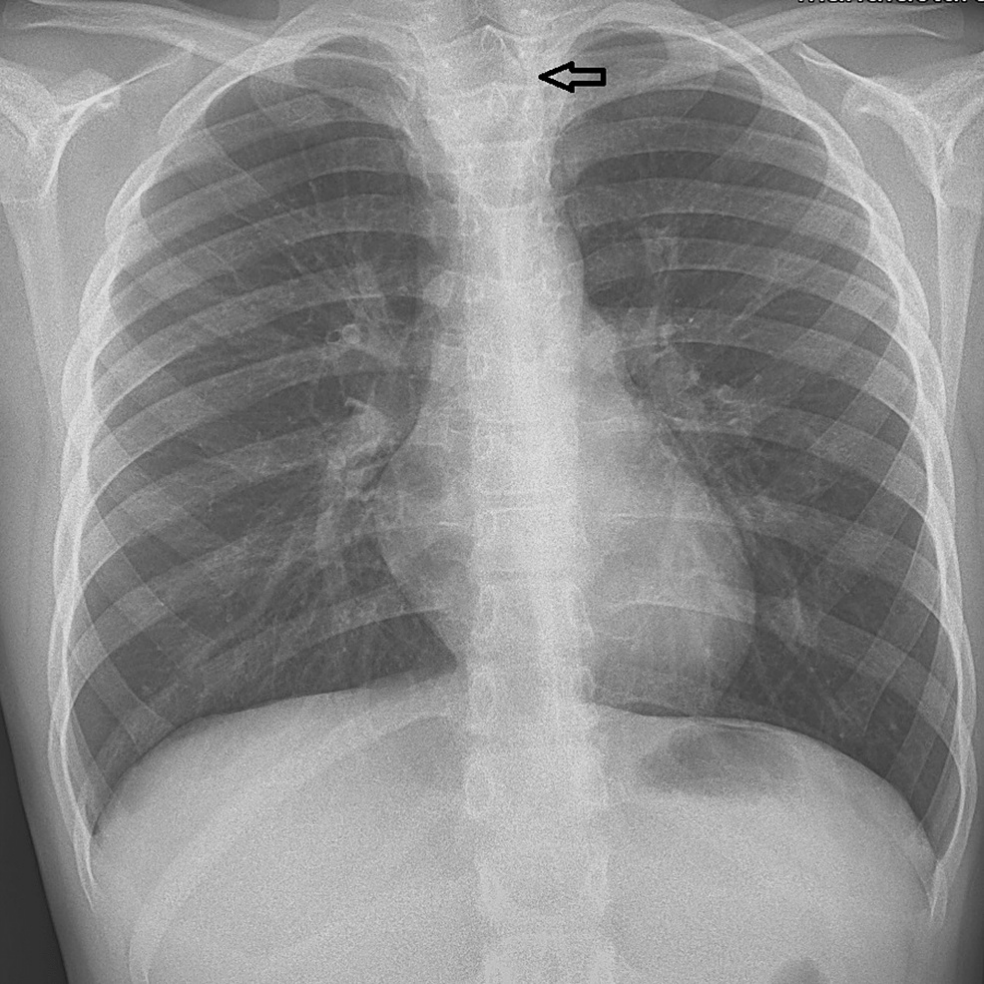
Chest X-ray A clear tracheal deviation can be seen in the chest X-ray.
